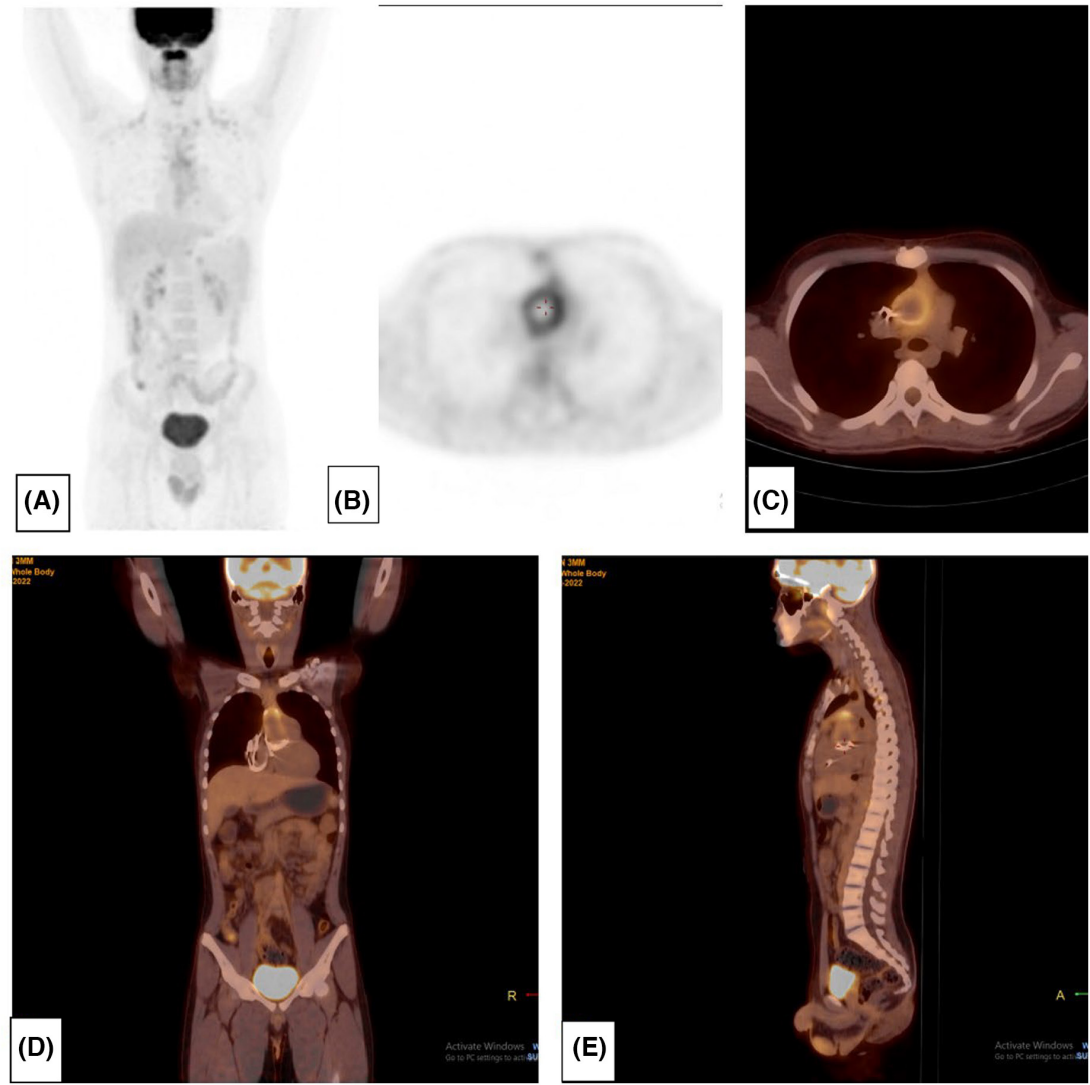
MIP (A), Trans‐axial PET (B), PET/CT (C), sagittal PET/CT (D) and Coronal PET/CT (E) images, showing increased 18F‐FDG uptake around the aortic root, that represented graft infection of the aorta.
